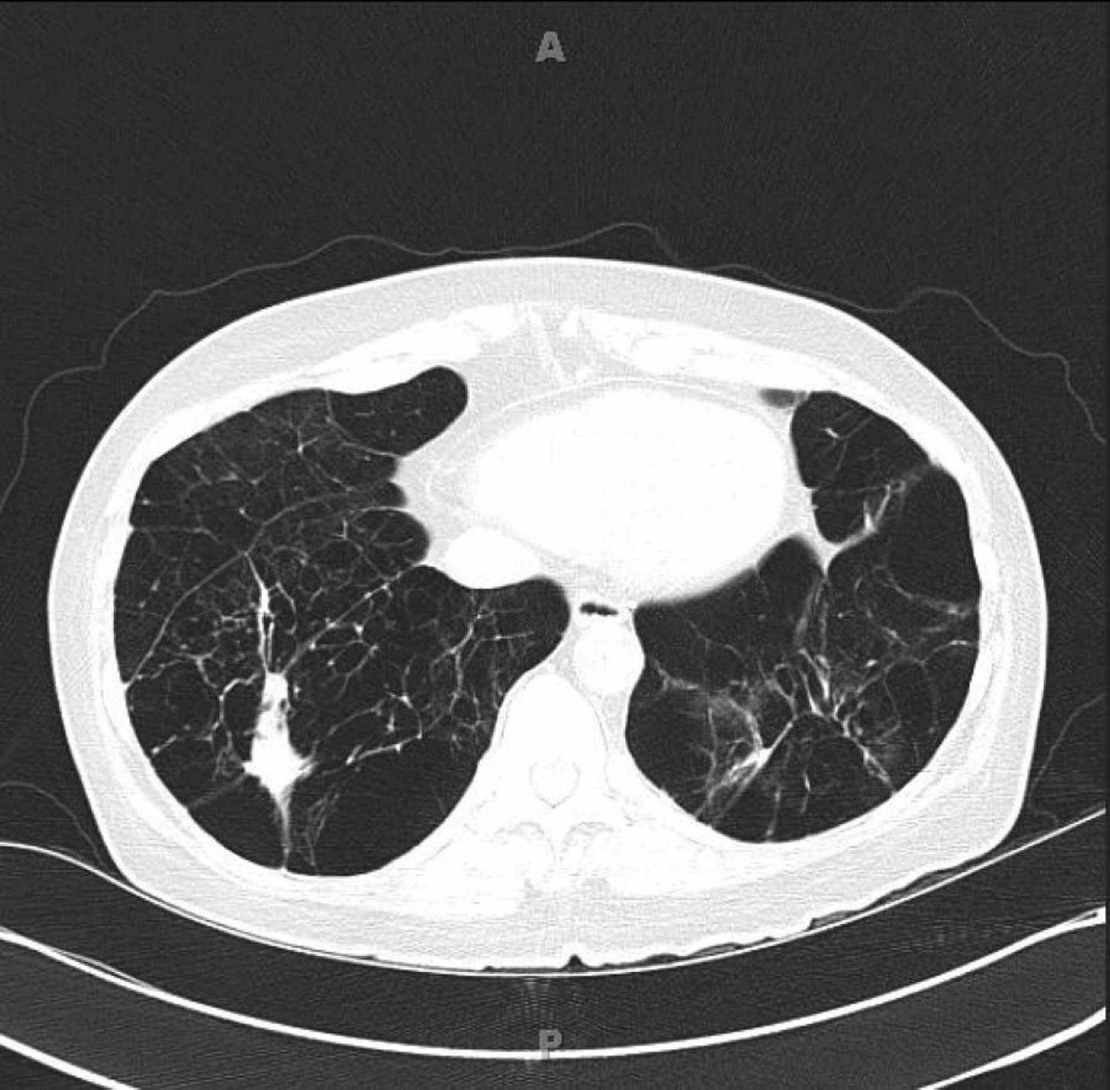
CT of the chest six months later Right lower lung mass is still present but stable in size. Chronic effects from alpha-1 antitrypsin deficiency including known bronchiectatic changes and severe bullous disease of the lower lobes were seen.
